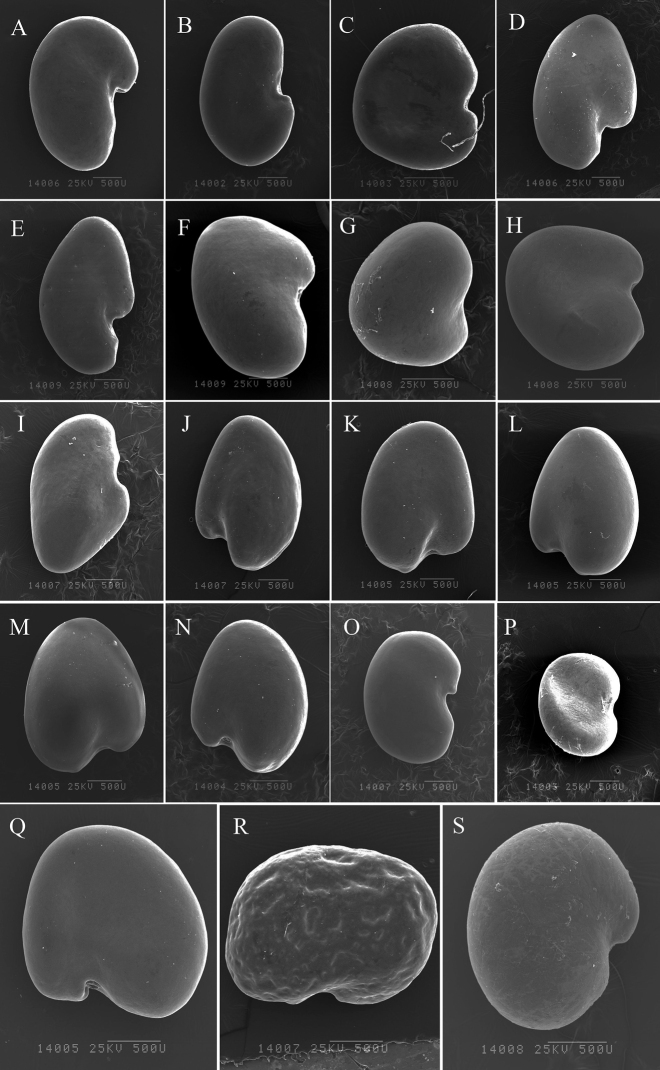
Seed shape of the studied species A*O.coerulea* (TT) B*O.coerulea* (EDG) C*O.holanshanensis*D*O.xinglongshanica* (MX) E*O.xinglongshanica* (XL) F*O.glabra*G*O.kansuensis* (AZ) H*O.kansuensis* (CT) I*O.taochensis*J*O.ochrocephala* (NH) K*O.ochrocephala* (XL) L*O.ochrocephala* (MX) M*O.ochrocephala* (JQ) N*O.ochrocephala* (HG) O*O.qinghaiensis*P*O.latibracteata*Q*O.qilianshanica*R*O.aciphylla*S*O.squammulosa*. Scale bar: 500 μm.
